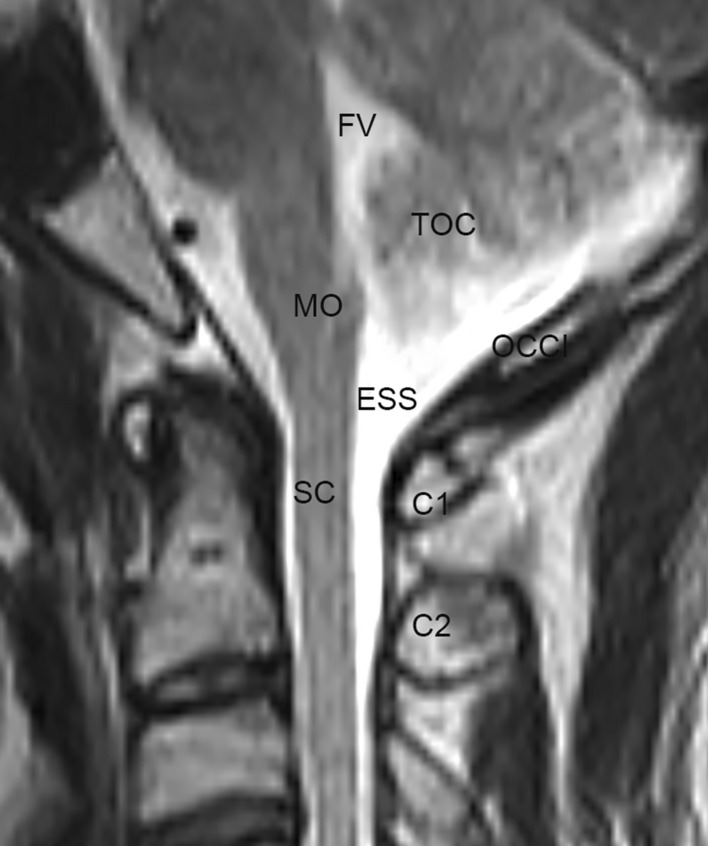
A median sagittal section of the head and neck (MRI). It was observed that the enlarged subarachnoid space was present on the dorsal side of the lower part of the medulla oblongata (MO) and the upper part of the cervical portion of the spinal cord (SC) and from the level of the foramen magnum down to the level of the axis (C2). OCCI, the occipital bone and the foramen magnum; C1, the posterior arch of the atlas; FV, fourth ventricle.
